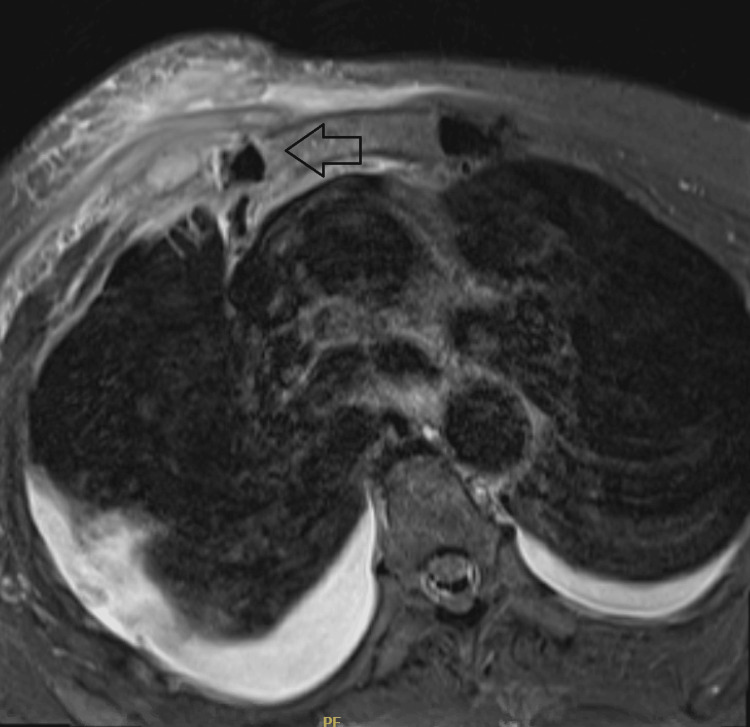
Preoperative right SCJ MRI showing large intra-articular joint effusion, with a collection deep to pectoralis major insertion and cutaneous reactions suggestive of an infective process (arrow pointing to findings). SCJ: sternoclaviclar joint
